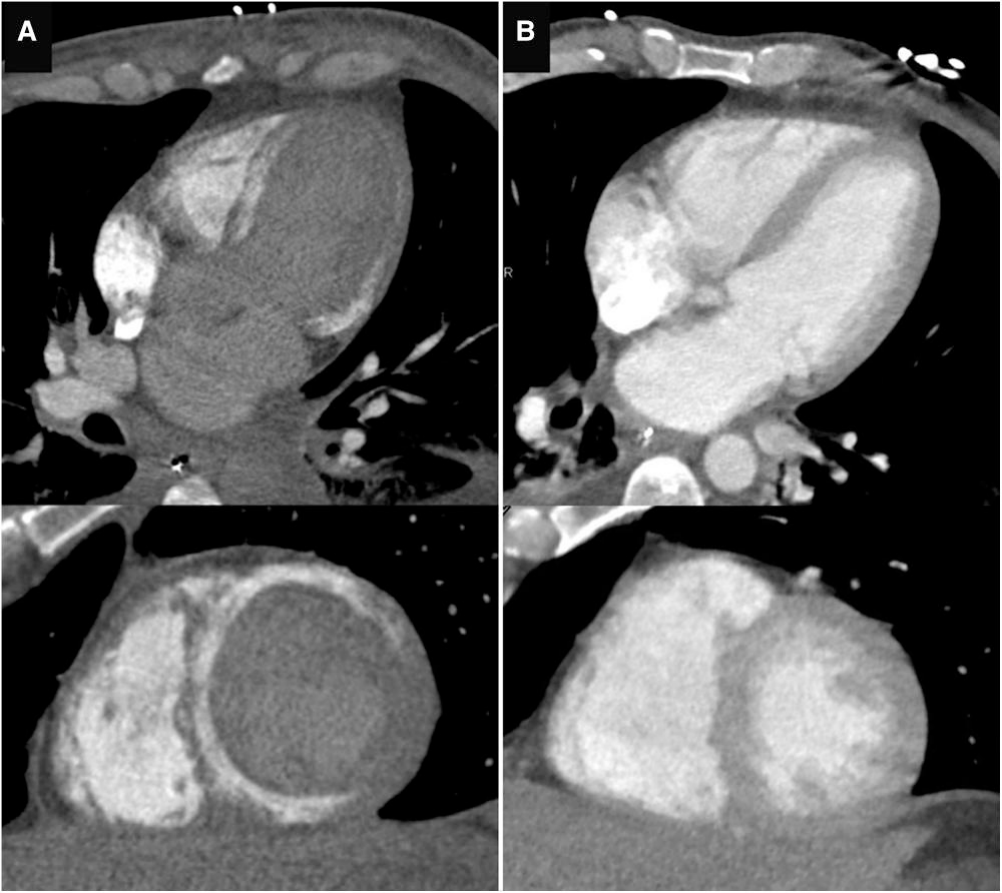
Multiplanar reformats of a chest CT after in-hospital cardiac arrest showing diffuse myocardial calcifications (*A*), compared with the normal chest CT performed 14 days earlier (*B*).
